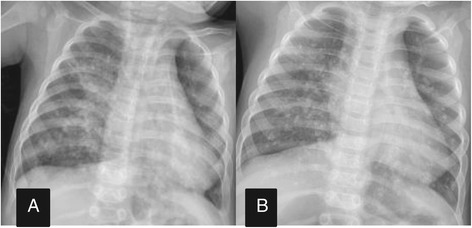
Chest radiography. A: A follow-up chest radiograph showed opacities in the bilateral lung remaining at 12 months of age. B: Chest radiography at 15 months showed nearly complete resolution of opacities with residual extensive spots of calcification.
